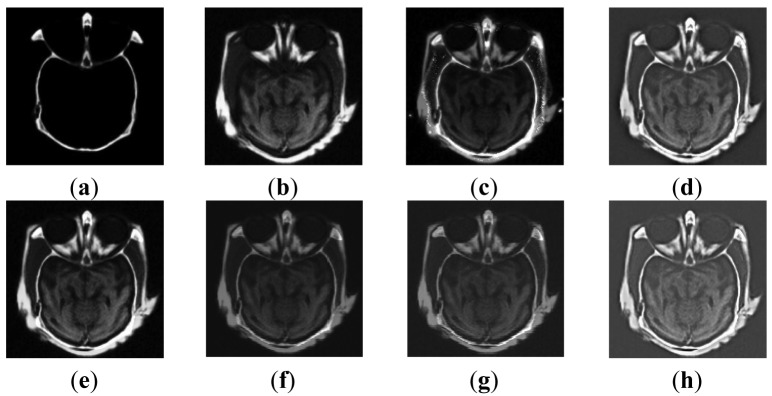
Medical source images and fusion results. (a) CT image; (b) MRI image; (c) Fused image based on M1; (d) Fused image based on M2; (e) Fused image based on M3; (f) Fused image based on PCA; (g) Fused image based on wavelet; (h) Fused image based on our method.
